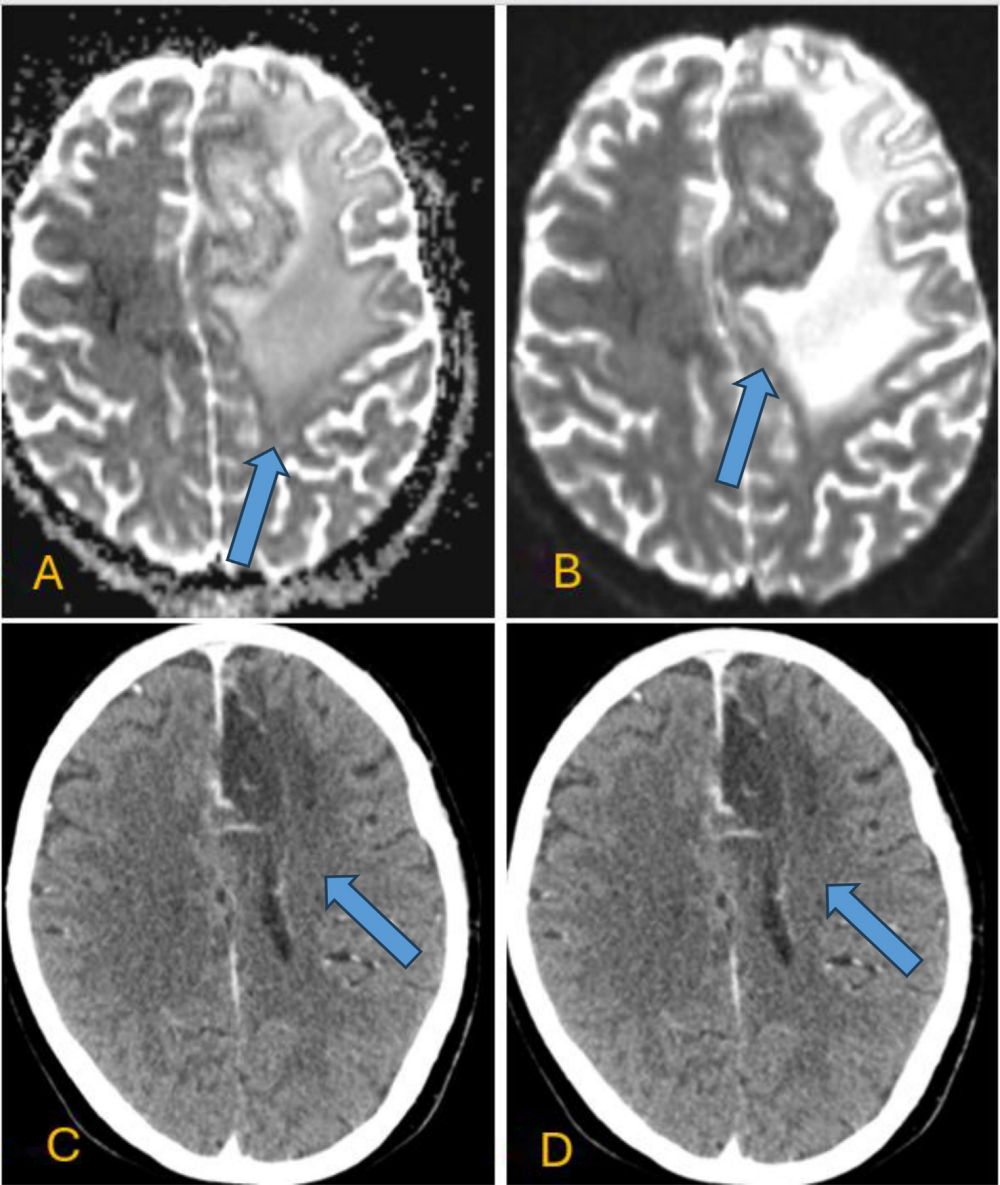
(A–B) Axial diffusion-weighted imaging (DWI) with corresponding ADC maps showing no diffusion restriction. (C–D) Axial plain CT scan (C) and axial post-contrast CT scan (D) of the brain following tumor resection, demonstrating a persistent ring-enhancing lesion in the left frontal lobe extending to the parafalcine region and crossing the midline. There is associated mass effect on the anterior horn of the left lateral ventricle, as well as the genu and body of the corpus callosum, with mild surrounding vasogenic edema.
